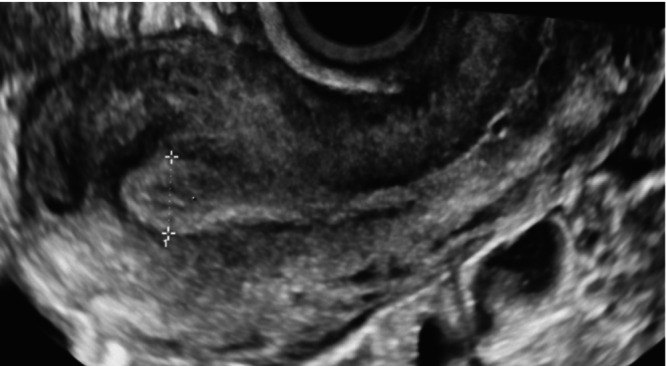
Example of double‐layer measurement of endometrial thickness on ultrasound (sagittal section). Calipers should be placed on the two opposite endometrial–myometrial interfaces in an appropriately magnified image (with the full length of the endometrial cavity visible and the uterus filling more than half of the screen), and the endometrium measured at the point at which it appears to be thickest, measuring perpendicular to the endometrial midline.
